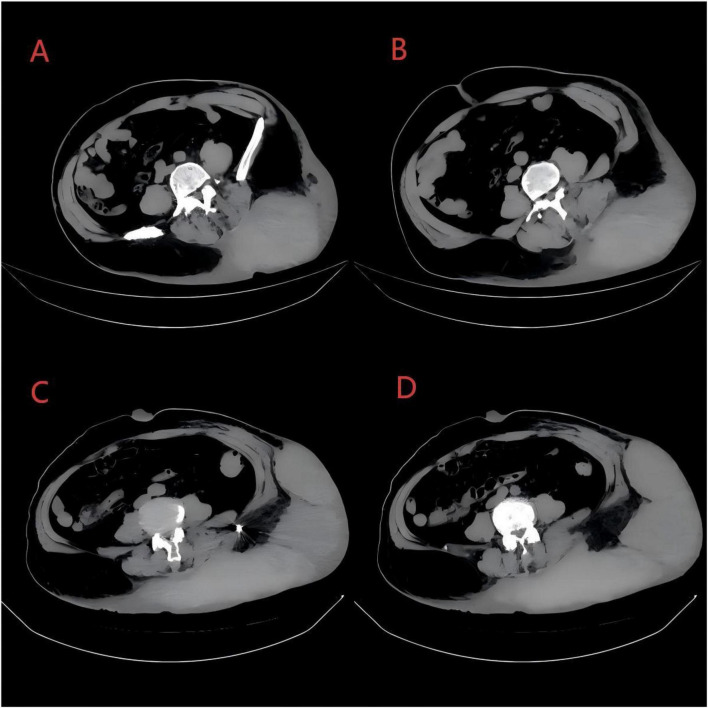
(A,B) Abdominal CT: there is marked skin thickening over the lower back and left gluteal region. A slightly hyperdense subcutaneous mass is noted on the left side of the lumbar region, suggestive of a possible hematoma. Contrast-enhanced imaging is recommended. A pre-sacral occupying lesion is identified, with extensive, multiple cutaneous nodules observed within the scanned field. (C,D) Post-embolization follow-up abdominal CT: compared to the prior lower abdominal imaging, the extent of the lesion appears to have increased, with a dense subcutaneous shadow observed on the left side of the lumbar region.
